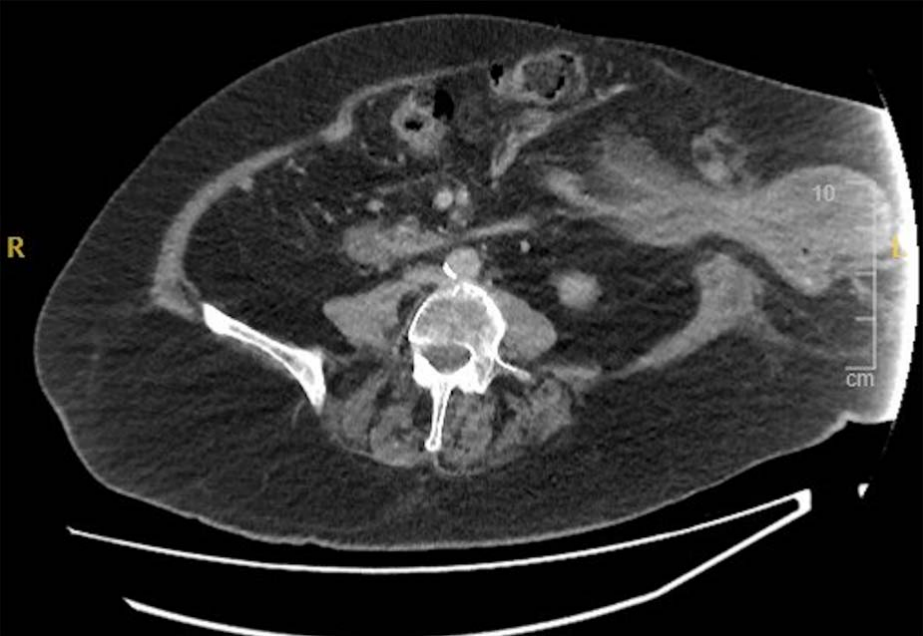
CT abdomen pelvis—axial view of parastomal hernia containing stomach.
